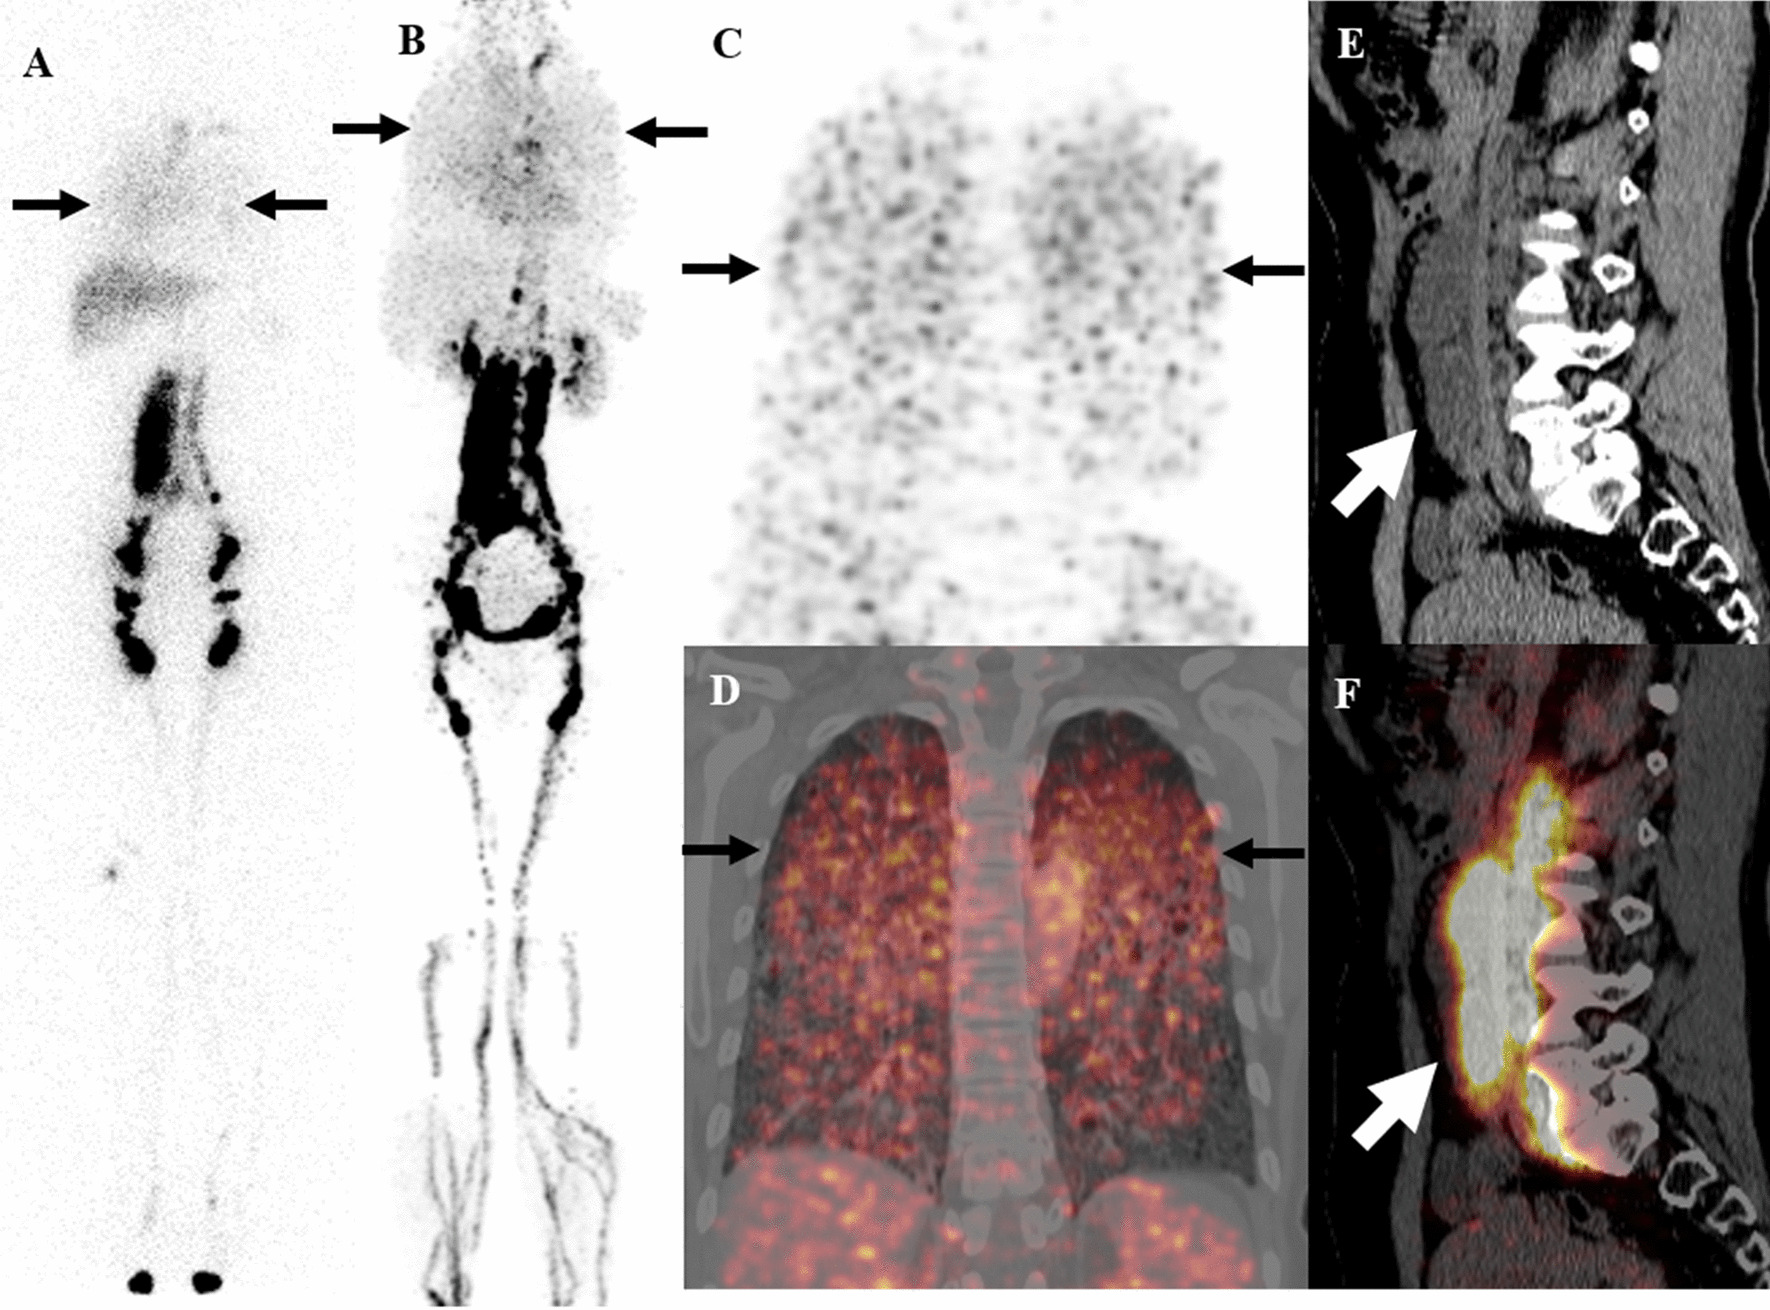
A 50-year-old woman (patient #2) with LAM. Both ^99m^Tc-ASC lymphoscintigraphy (A, black arrows) and ^68^Ga-NEB PET/CT MIP image (B, black arrows) showed diffuse, increased activity in the chest and intense vertical activity in the abdomen. Coronal images (C, PET; D, fusion; black arrows) of ^68^Ga-NEB PET/CT of the chest demonstrated that the abnormal chest activity was diffusely distributed in the lung, suggesting diffuse pulmonary lymphatic changes. On sagittal images (E, CT; F, fusion; white arrows) of ^68^Ga-NEB PET/CT of the abdomen, the vertical activity was located in the dilated lumbar trunk
